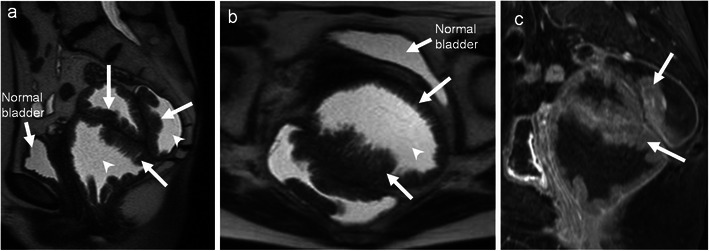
A 61 year-old-man presenting to the emergency department with severe dehydration resulting from intermittent episodes of diarrhoea and profuse mucous discharge from the rectum, found to have villous adenoma. a Sagittal T2-weighted MRI and b axial T2-weighted MRI reveal a carpet-like “hairy” lesion covering the mucosa of the rectum (*arrows*). The lumen of the rectum is distended and fluid filled (*arrowhead*) with no proximal obstruction. c Contrast-enhanced MRI reveals enhancement of the thickened abnormal mucosa (*arrows*)
